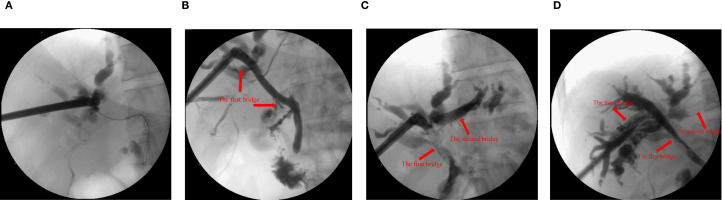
X-ray examination with contrast agent injected through the sinus. (A) the common bile duct before guide wire puncture and balloon dilation. (B) the right anterior intrahepatic bile duct and the common bile duct were bridged with metal stent (10x60 mm, the first bridge). (C) the left and right anterior intrahepatic bile ducts were bridged with metal stent (8x60 mm, the second bridge). (D) the right anterior and right posterior intrahepatic bile ducts were bridged with metal stent (8x60 mm, the third bridge).
